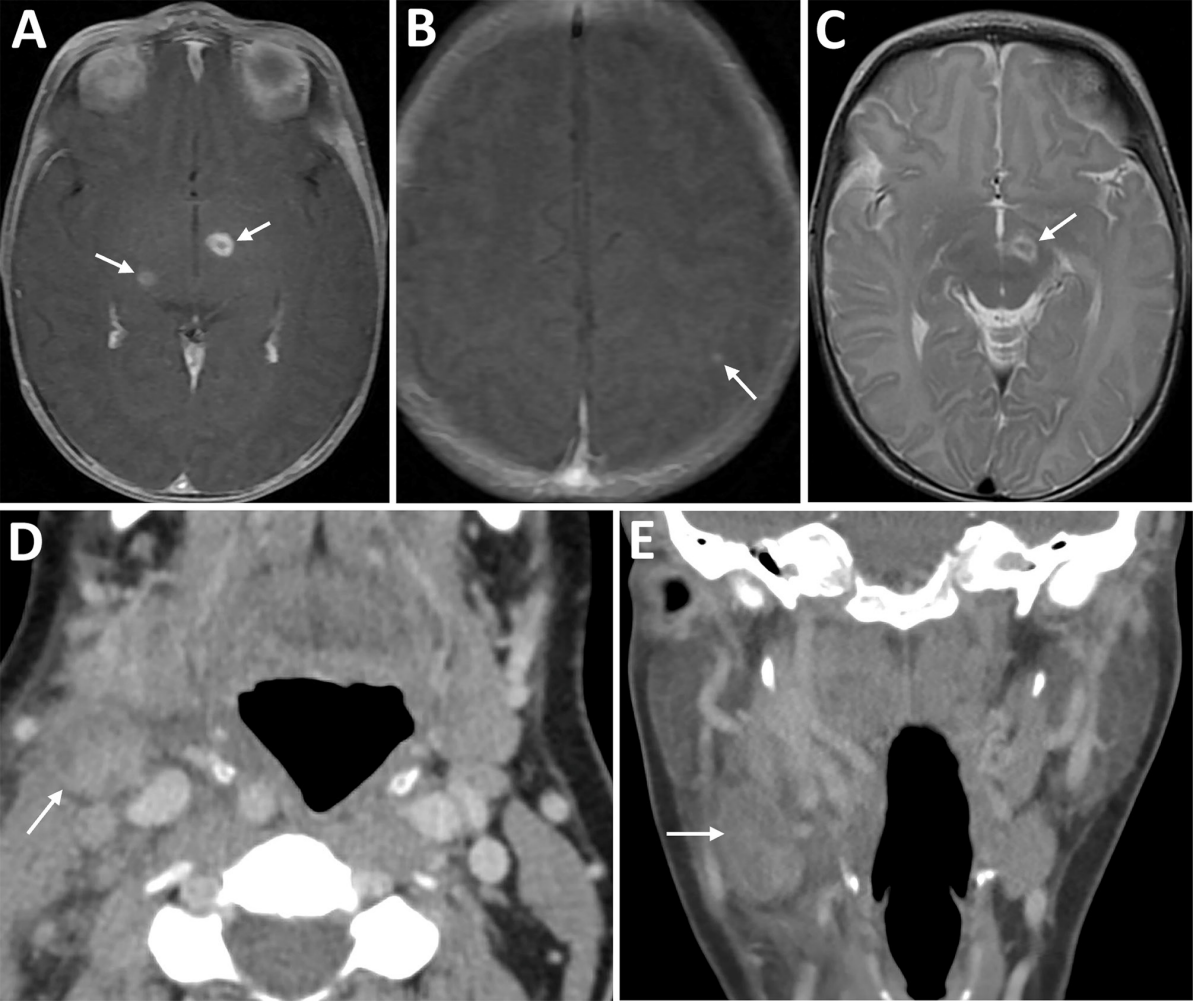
Imaging from infant and mother in case of congenital tularemia with neuroinvasive disease, Utah, USA. A, B) Axial T1 post-contrast images showing the infant’s initial magnetic resonance imaging findings of rim enhancing lesions near the left subthalamic nucleus and right inferior thalamus (arrows, panel A), as well as a punctate enhancing lesion in the left parietal lobe (arrow, panel B). C) Axial T2 image demonstrating T2 hyperintense edema along the margins of the largest lesion near the left subthalamic nucleus (arrow). D, E) Axial (D) and coronal (E) images from the mother’s computed tomography scan with intravenous contrast showing an enlarged, heterogeneous right cervical chain lymph node with inflammatory stranding in the adjacent soft tissues (arrows).
